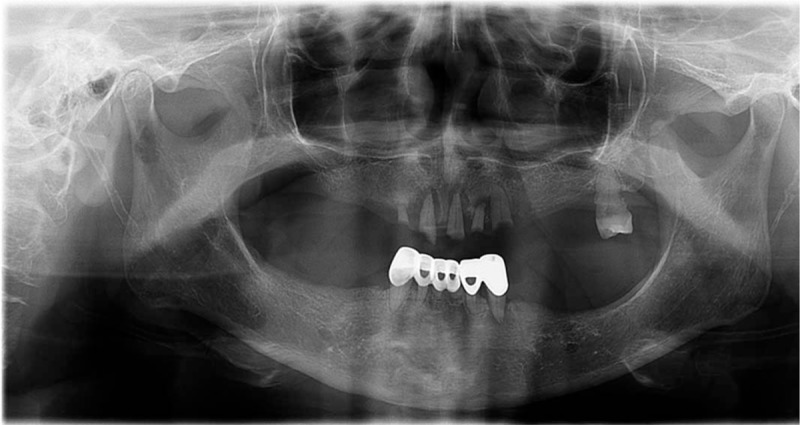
Jaw X-ray. The jaw X-ray showed that the patient had several missing teeth (April 11, 2017).
